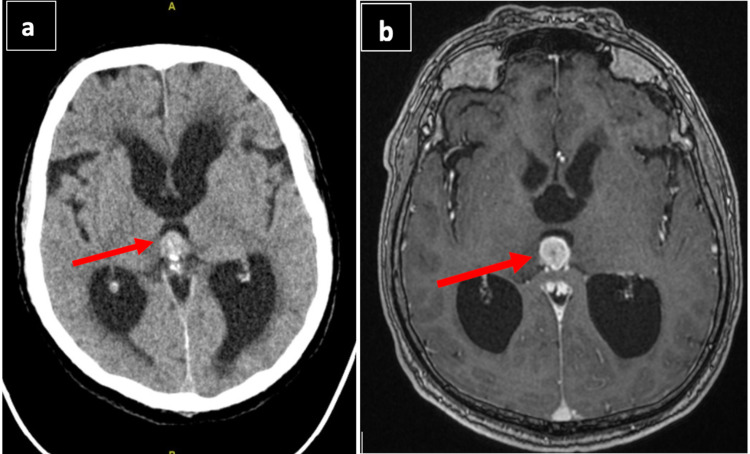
a) Plain CT scan of the brain axial view showing a hyperdense mass in the pineal region obstructing the posterior third ventricle with active hydrocephalus (arrow). b) MRI of the brain with contrast showing the same pineal region mass, hyperintense in looks, measuring 1.6x1.3x1.6 cm obstructing the posterior third ventricle with active hydrocephalus (arrow).
